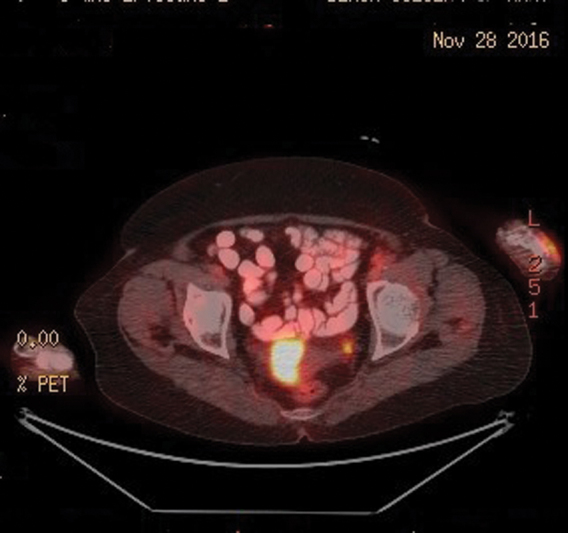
FDG-PET/CT showed a mass filling the uterine cavity and metastatic lymph node (SUV: 9.1). CT, computed tomography; FDG, fluorodeoxyglucose; PET, positron emission tomography.
